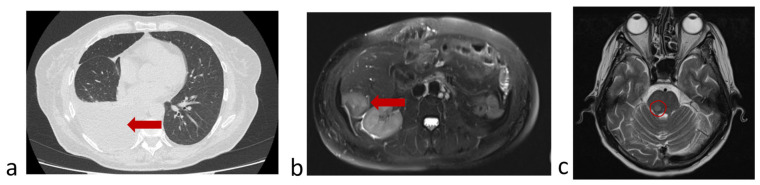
Protocol code 13: (a) abdomen MRI from 26 October 2022: the liver structure is heterogeneous due to focal lesions of different sizes (*n* = 6) with unclear uneven contours measuring 4.1 × 3.7 (previously 4.0 × 3.0 cm) with signs of contrast accumulation; (b) chest CT from 28 October 2022: Pneumatization of the upper right lobe is reduced. Fluid in the right pleural cavity is up to 64 mm thick (previously 16 mm) with a volume of up to 1750 mL; (c) brain MRI from 29 October 2022: in the substance of the brainstem on the right, a formation measuring 7 × 5 mm with accumulation of contrast is determined (marked with a circle).
